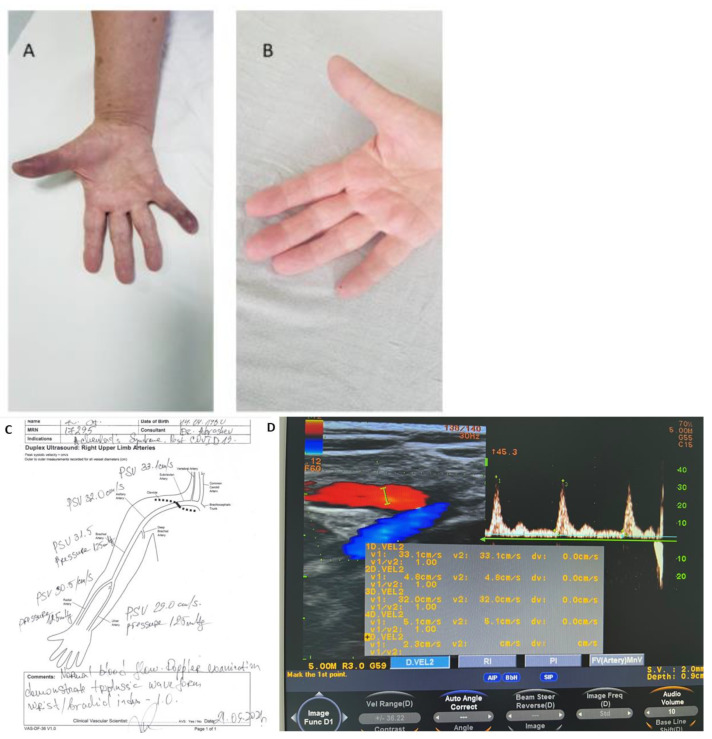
(A,B). A 71-year-old non-smoking woman presented with sudden swelling and bruising along the volar aspect of her first and fifth fingers on her right hand. Hand pictures: (A) the first day of admission; and (B) the first day of discharge. (C,D). Data of Doppler investigation (C) and Doppler ultrasound result (D) showed normal peak forward velocities in cm/s. Doppler examination demonstrates triphasic waveforms. Brachial/wrist index of 1.0.
